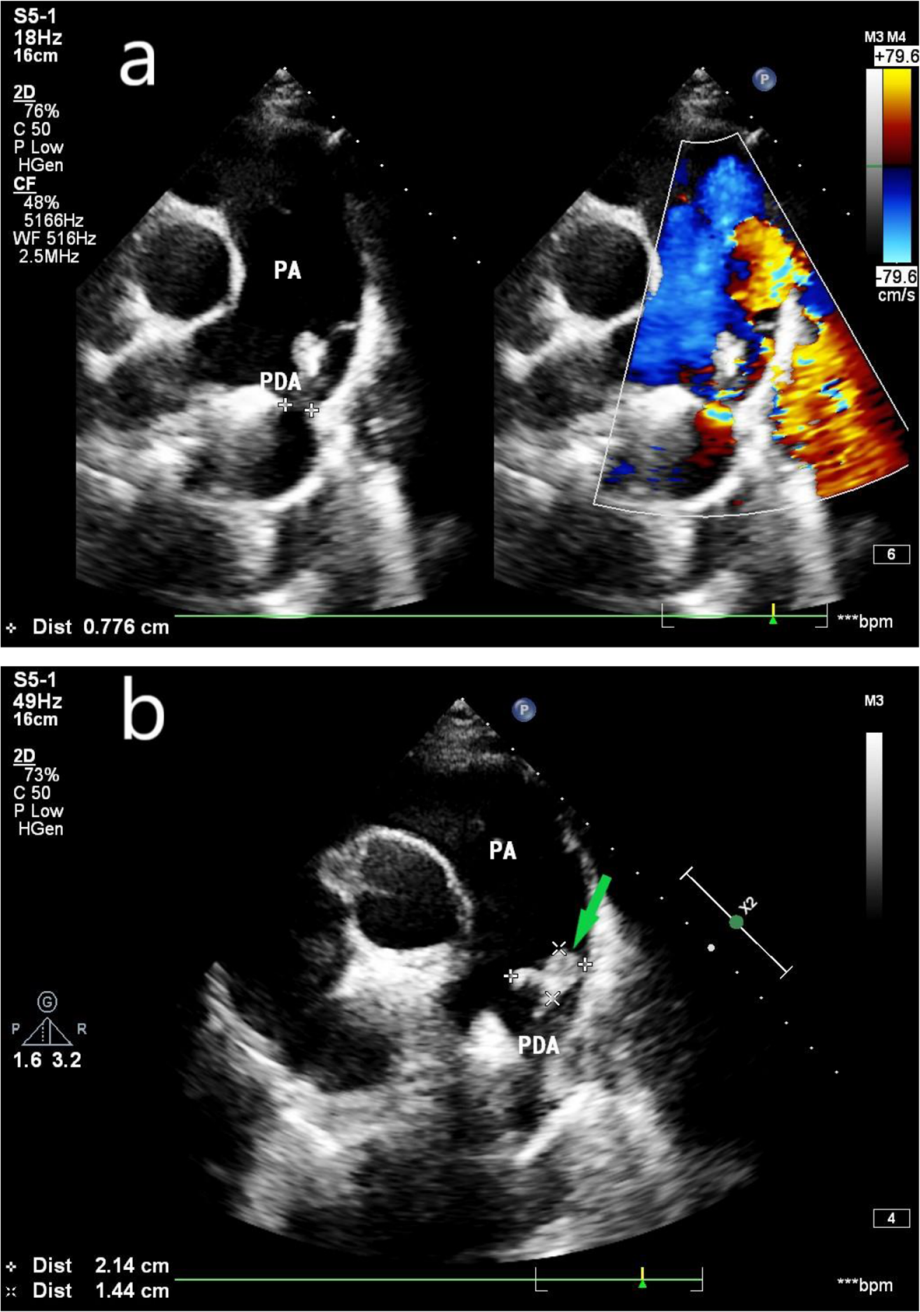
a Echocardiography at 26 weeks of gestation shows patent ductus arteriosus between the descending aorta and left pulmonary artery; b at the opening of the PDA, there is a vegetation (PA = pulmonary artery; PDA = patent ductus arteriosus; white arrow = infective endocarditis vegetative)
